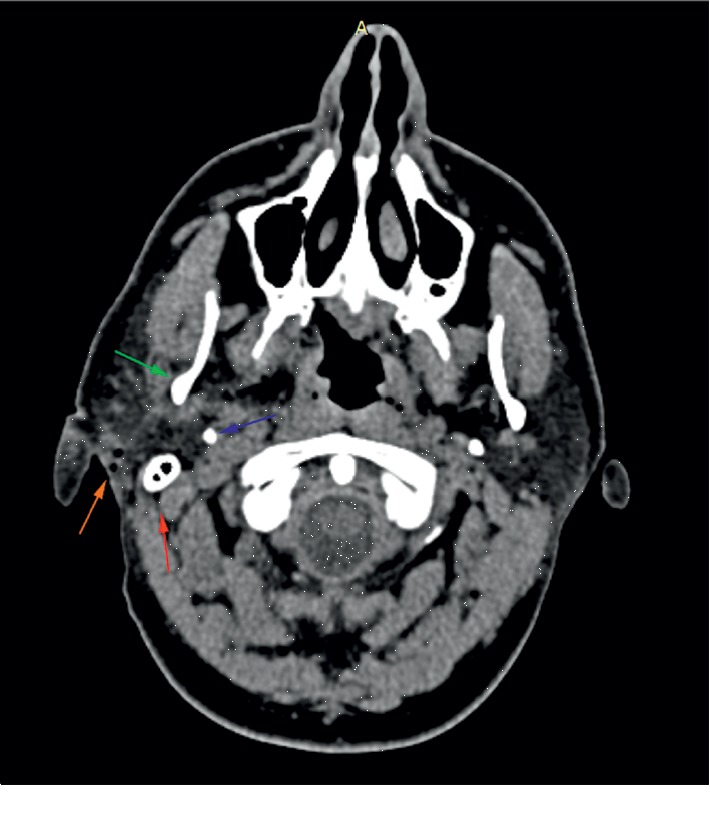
CT scan showing a mastoid tip (red arrow), styloid process (blue arrow), ramus of the mandible (green arrow), and entry trajectory (orange arrow).
